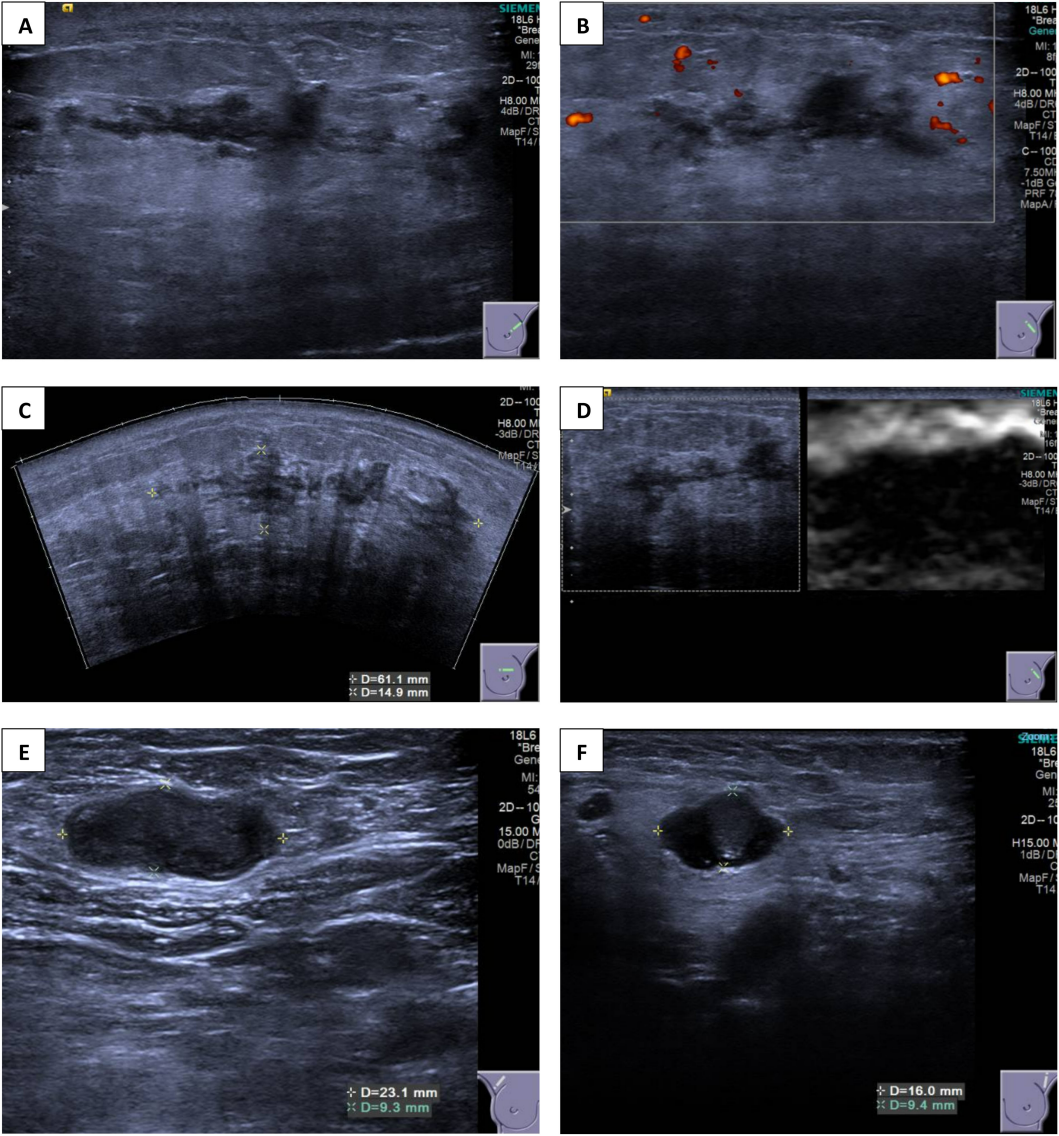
(A–D) Ultrasound images of the left breast showing multiple ill-defined heterogeneously hypoechoic lesions extending from the 11 o’clock to 3 o’clock position. (E, F) Ultrasound images of bilateral axilla showing abnormal axillary lymphadenopathy.
